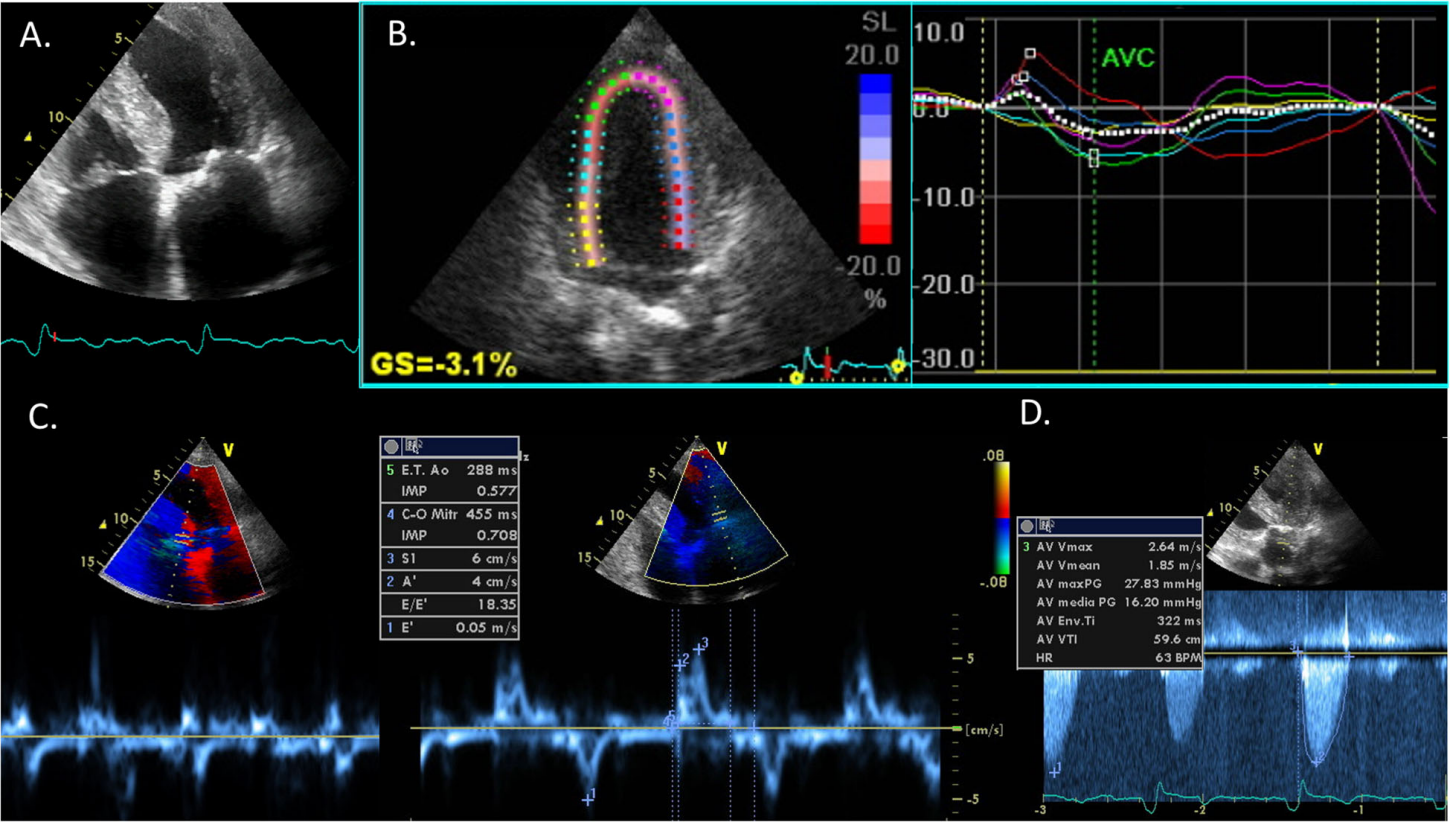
a Transthoracic echocardiographic apical 4-chamber view showing severe LV hypertrophy with myocardial granular sparkling, biatrial dilatation and atrial septal thickening. b Longitudinal strain analysis revealing severely impaired longitudinal systolic function without apical sparing. c Lateral and septal mitral annular tissue Doppler showing a severely reduced average S’. d Continuous wave doppler across LV outflow tract showing low gradient AS
